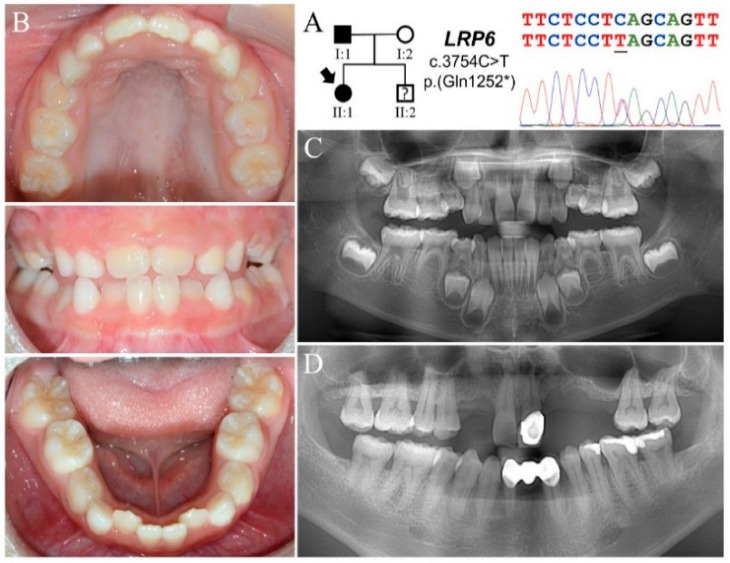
Family 1 with a novel *LRP6* p.(Gln1252*) mutation. (A) The Family 1 proband (II:1) inherited FTA from her father (I:1), suggesting a dominant pattern of inheritance. The DNA sequencing chromatogram shows a C-to-T transition that converts a CAG (glutamine) codon into a TAG (stop) codon in one *LRP6* allele. (B) The proband, age seven, presented with a mixed dentition with no apparent microdontia or tooth dysmorphology. All primary first molars appeared to be in infraocclusion. (C) The panoramic radiograph of the proband revealed a total of eight missing tooth germs excluding third molars. Taurodontism of primary and permanent molars was not evident. (D) The father had multiple missing teeth and wore a maxillary partial denture. While tooth numbers 6, 11, and 12 were previously extracted, tooth numbers 7, 10, 13, 23, 24, and 25 as well as all third molars were congenitally absent.
